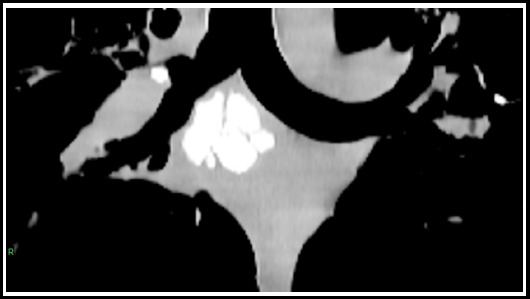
CT scan chest in mediastinal window setting shows multiple calcified subcarinal nodes. A small calcified node is seen adjacent to the main right bronchus which can erode the bronchus and result in a broncholith.
